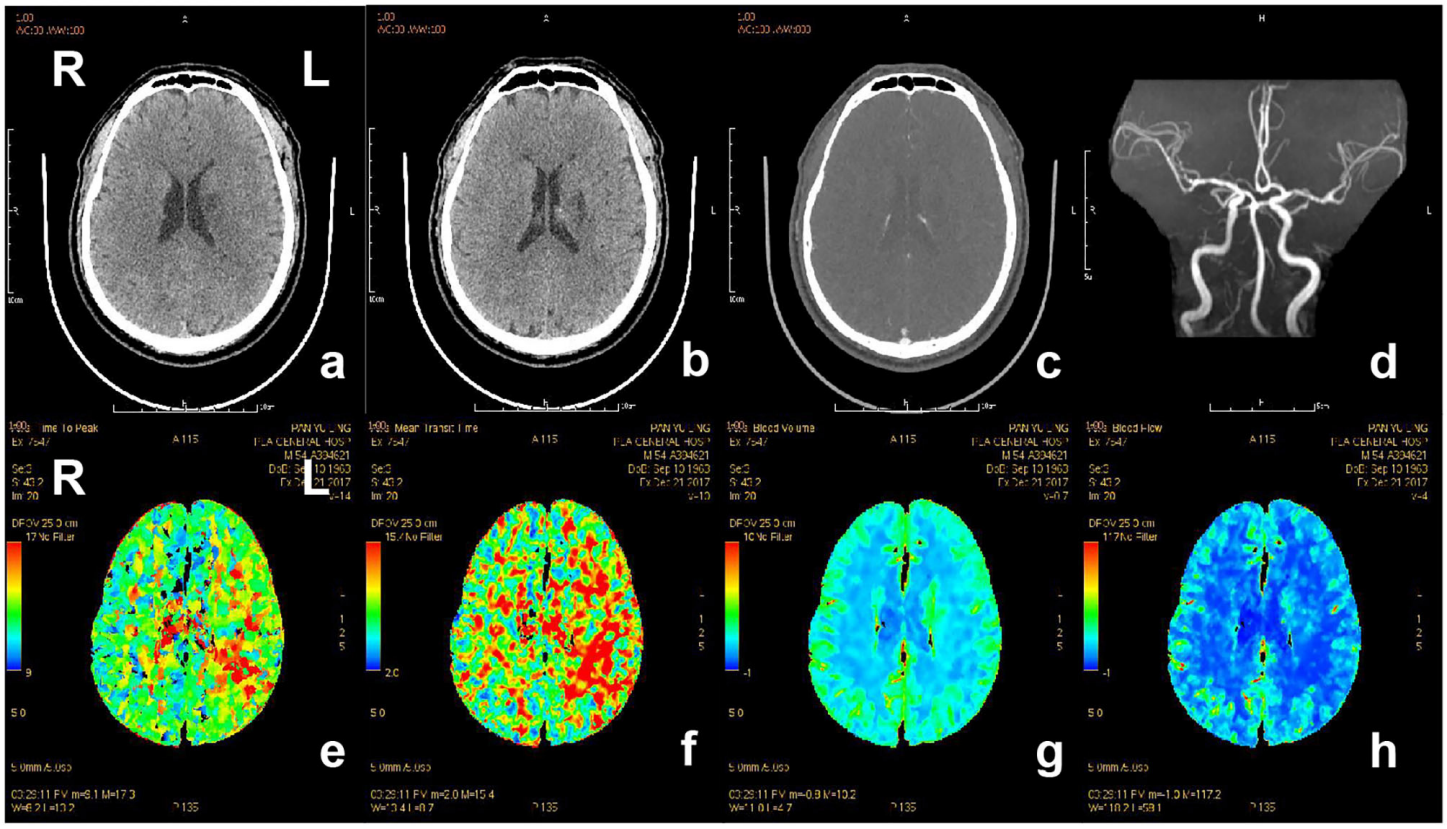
CT and CTP imaging of the patient with acute ischemic stroke. The patient, 80-year-old-female, suffered from weakness of right limbs, alalia and was unable to walk 3.5 h prior to the imaging. Medical history included hypertension, atrial fibrillation and gout. Physical examination showed right facial paralysis, the muscle strength of right limbs was grade I, right Babinski sign (+), and NIHSS score was 10. The diagnosis of the patient is acute ischemic stroke. The patient was given multimodal CT and MRA before recanalization. a and b, The left bilateral basal ganglia and bilateral frontal lobe showed sporadic dots and patches with low-density shadow on non-contrast CT scan. c, Contrast-enhanced CT showed no abnormal enhanced shadow. d, MRA showed the lumen of bilateral middle cerebral artery was not smooth, the left cerebral artery M1 segment had multiple stenosis, and distal branch vessels were disappeared. e and f, Prolonged TTP and MTT in the area of left middle cerebral artery. g and h, CBF and CBV showed no significant abnormal area. CT, computed tomography; CTP, computed tomography perfusion; CBV, cerebral blood volume; CBF, cerebral blood flow; TTP, time to peak; MTT, mean transit time.
